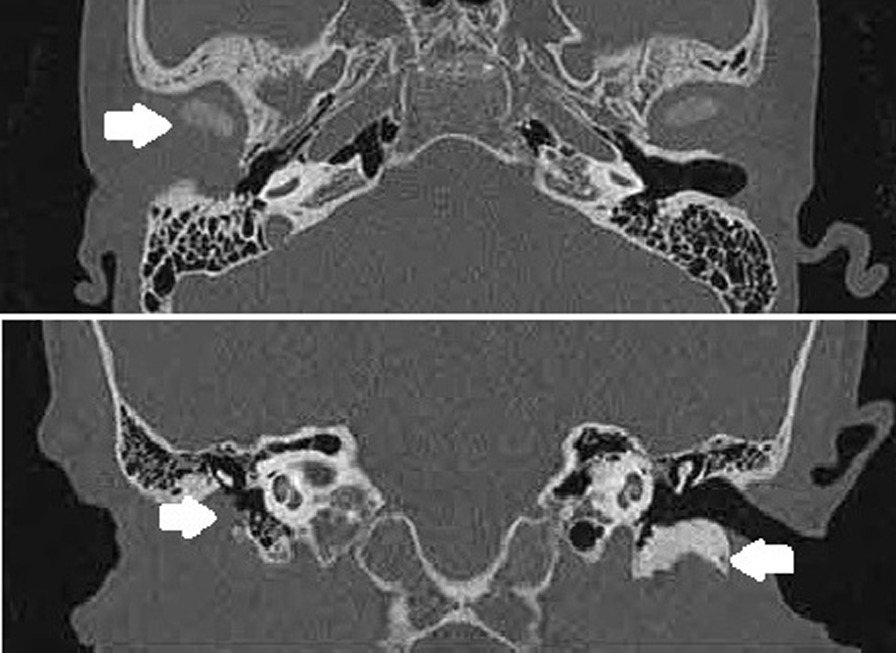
Temporal bone HRCT of patient No. 13 in the axial and coronal sections. Right arrow, undeveloped tympanic portion of the temporal bone and retrodisplaced mandibular condyle; left arrow, normal tympanic portion of the contralateral ear
